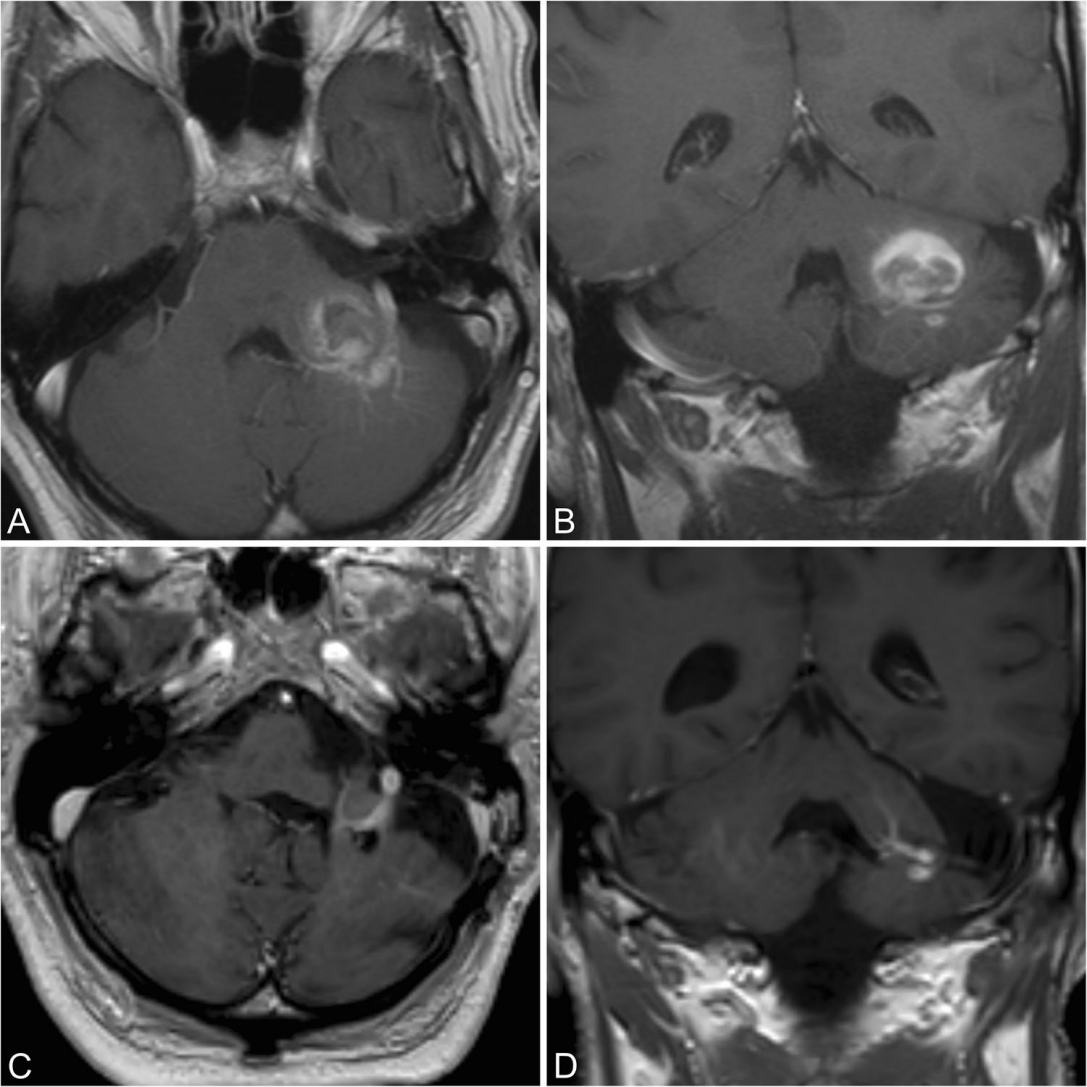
CM in the left middle cerebellar peduncle. Preoperative axial (a) and coronal (b) T1-weighted images with gadolinium demonstrating cavernoma with associated DVA. Postoperative axial (c) and coronal (d) T1-weighted images with gadolinium, showing complete resection of the CM with preservation of the DVA
